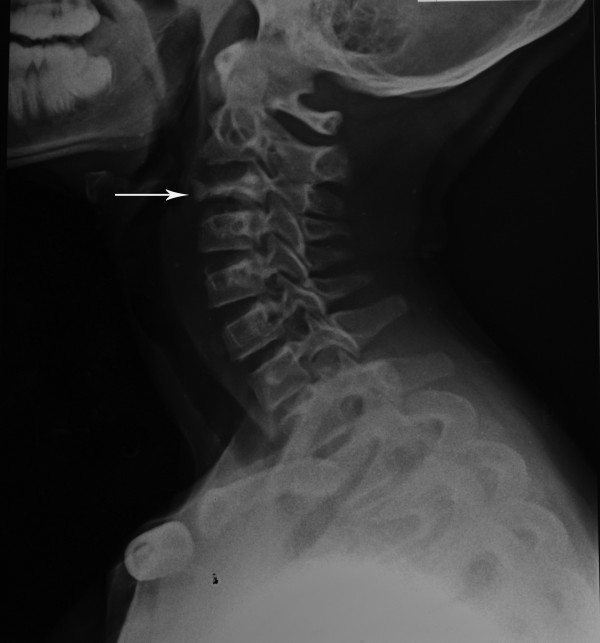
Radiograph taken at end of treatment showing increased height and density of anterior portion of third cervical vertebra (arrow) and normal spine curvature.
